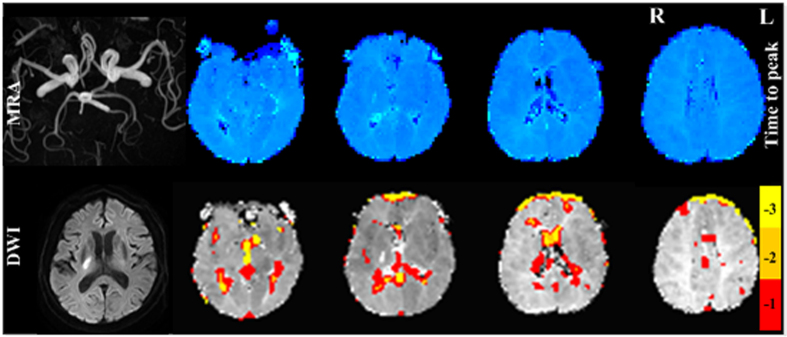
A single example patient among those who had no obvious MRA lesions, there were neither TTP delay regions nor time delay areas from TSA. MRA = magnetic resonance angiography, DWI = diffusion weighted imaging. −1, −2, −3 in color bar indicate −1TR, −2TR, −3 TR time shift.
